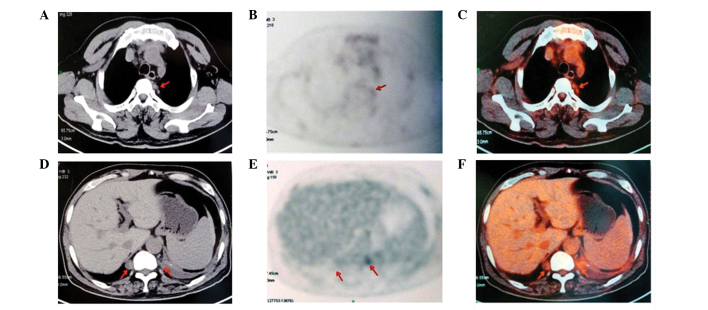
EMH at the posterior-superior mediastinum (T3-T4 vertebral level) and the posterior-inferior mediastinum (T10 vertebral level) on a postoperative PET/CT scan (red arrow). (A) CT image, (B) PET image and (C) fused image at T3-T4 vertebral level. PET/CT indicated slightly increased FDG uptake in mediastinal mass at the T3-T4 vertebral level. (SUV_max_, 5.2; SUV average, 2.8). (D) CT image, (E) PET image and (F) fused PET/CT image at the T10 vertebral level detected EMH as a benign-appearing mass. SUV_max_ was markedly decreased in EMH (SUV_max_, 3.8; SUV average, 2.4). EMH, extramedullary hematopoiesis; PET/CT, positron emission tomography-computed tomography; FDG, 18-fluorodeoxyglucose.
